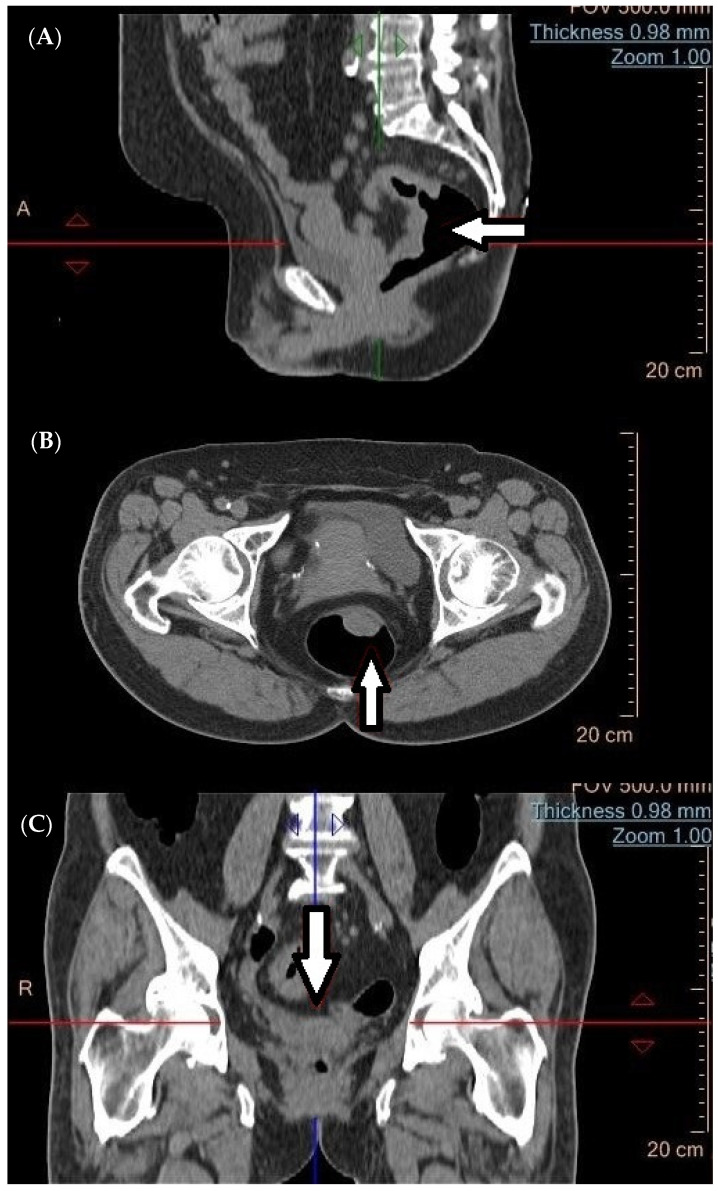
A rectal tumor (arrow) detected using CT in the (A) sagittal, (B) transverse, and (C) coronal planes, respectively.
